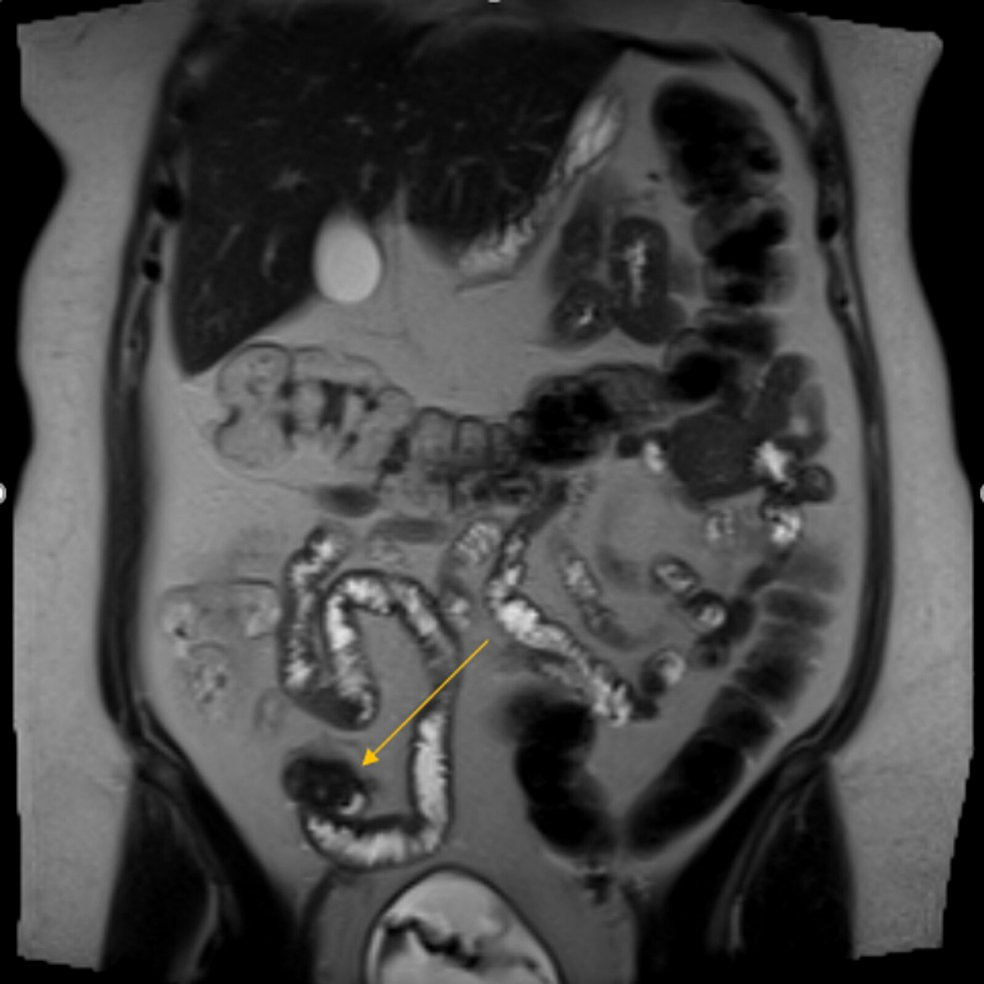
MRI abdomen T2 HASTE coronal view: image demonstrates an area of abnormal peri-enteric thickening in the distal ileum in the right iliac fossa. Differentials included Meckel's diverticulum and endometriosis of the small bowel.
